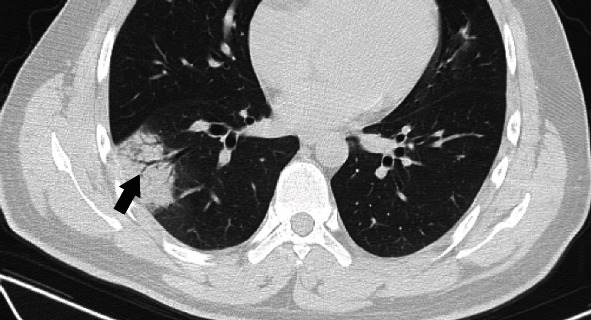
Imaging in a 26-year-old man who presented with fever for 6 days. Axial CT image shows patchy consolidation with air bronchogram (black arrow) in the lateral basal segment of the right lower lobe.
